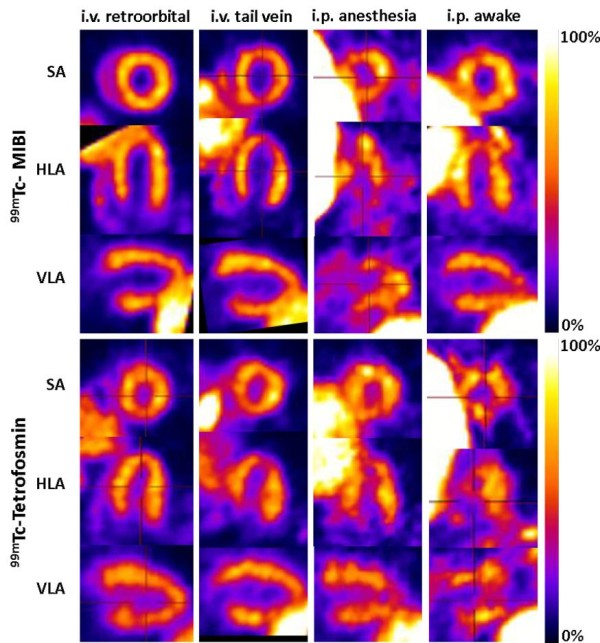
Examples of the eight different SPECT protocols for the best time frame. Examples of eight different SPECT protocols for the best time frame in midventricular short axis (SA), horizontal long axis (HLA) and vertical long axis (VLA). This figure demonstrates the good-excellent image quality of the i.v. protocols, whereas the i.p. protocols result in poor and variable image quality.
